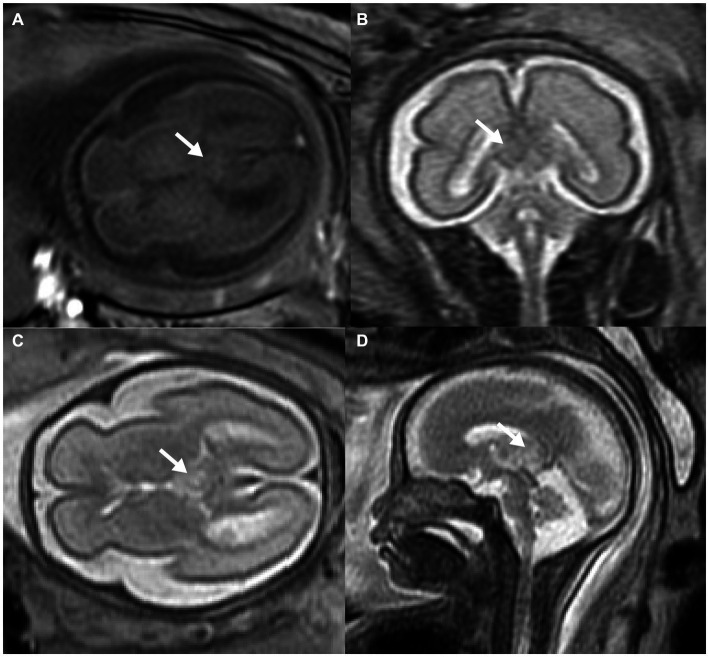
Prenatal MRI of fetal intracranial medulloepithelioma. MRI of the fetal brain: axial view of T1WI (A), coronal (B), axial (C), and sagittal (D) view of T2WI; white arrow indicates the tumor.
